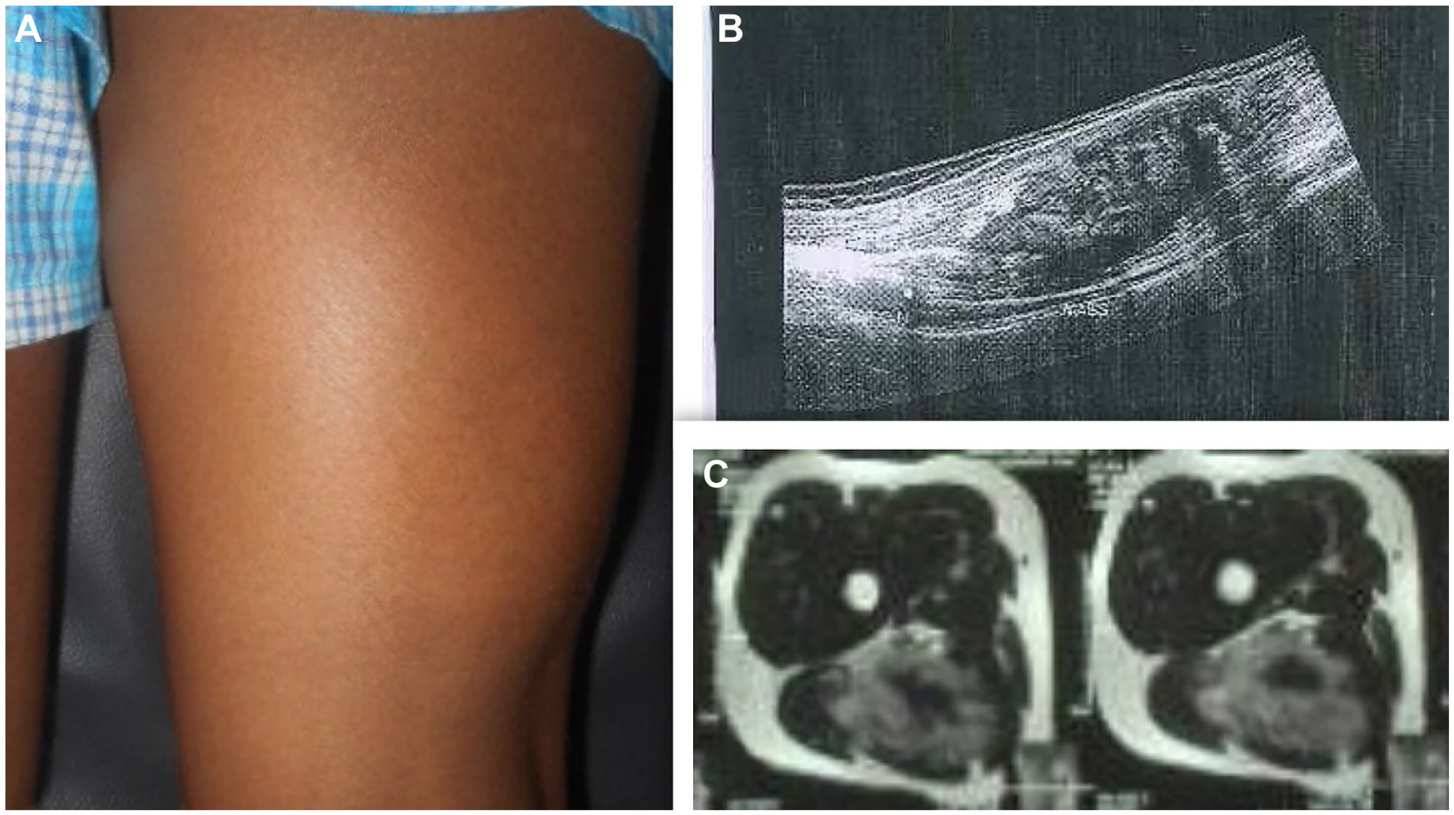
(A) Clinical picture, (B) Usg showing intramuscular heterogenous lesion arising from sciatic nerve, (C) MRI showing heterogeneously isointense mass measuring 12.8 × 6.6 × 3.8 cm with features suggestive of neurofibroma.
